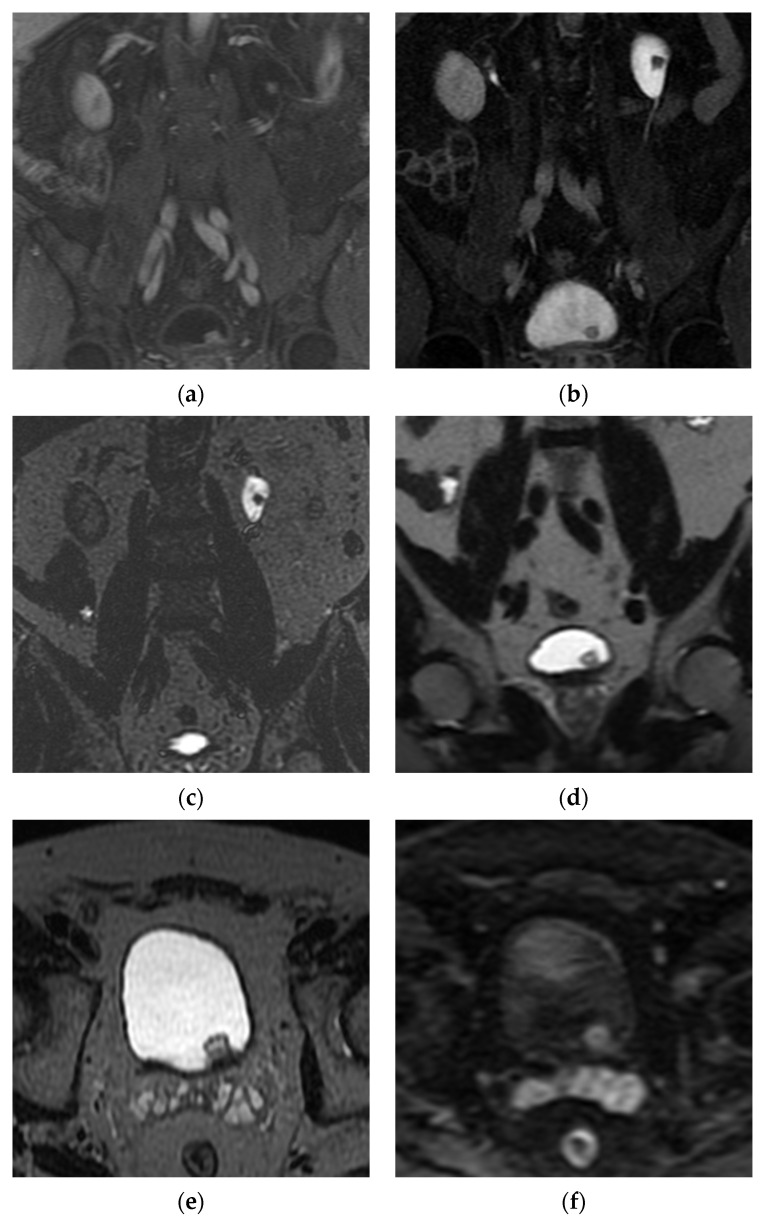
MR urography obtained in a patient with Lynch syndrome showing synchronous upper and lower tract lesions. Pathology of the bladder lesion confirmed non-invasive urothelial carcinoma and upper tract washings were concerning for low grade urothelial carcinoma. (a) Coronal post-contrast T1WI shows enhancing lesions in the bladder (solid white arrow) and left renal pelvis (dashed white arrow). (b) Coronal post-contrast excretory phase image shows the lesions as filling defects, surrounded by excreted contrast. (c) Coronal T2WI shows the upper tract lesion surrounded by T2 hyperintense urine. (d) Coronal T2WI of the bladder shows the bladder lesion as T2 intermediate in signal without invasion into the T2 hypointense muscularis propria. (e) Axial T2WI of the bladder shows the mass to be non-invasive. (f) Axial DWI shows restricted diffusion of the mass.
